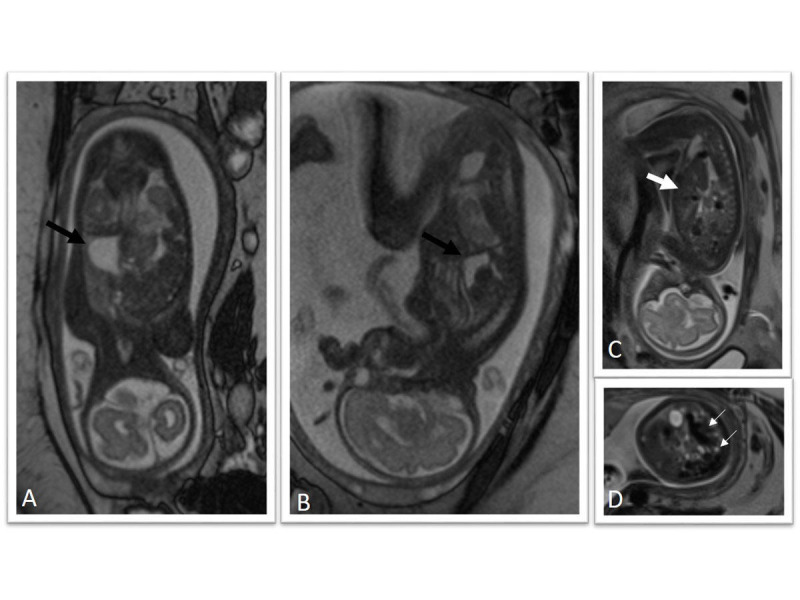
Congenital diaphragmatic hernia Coronal (A), sagittal (B and C), and axial T2-weighted HASTE images demonstrate a large left-sided diaphragmatic hernia containing stomach (black arrow), left hepatic lobe (thick white arrow), and loops of bowel (small white arrows).
